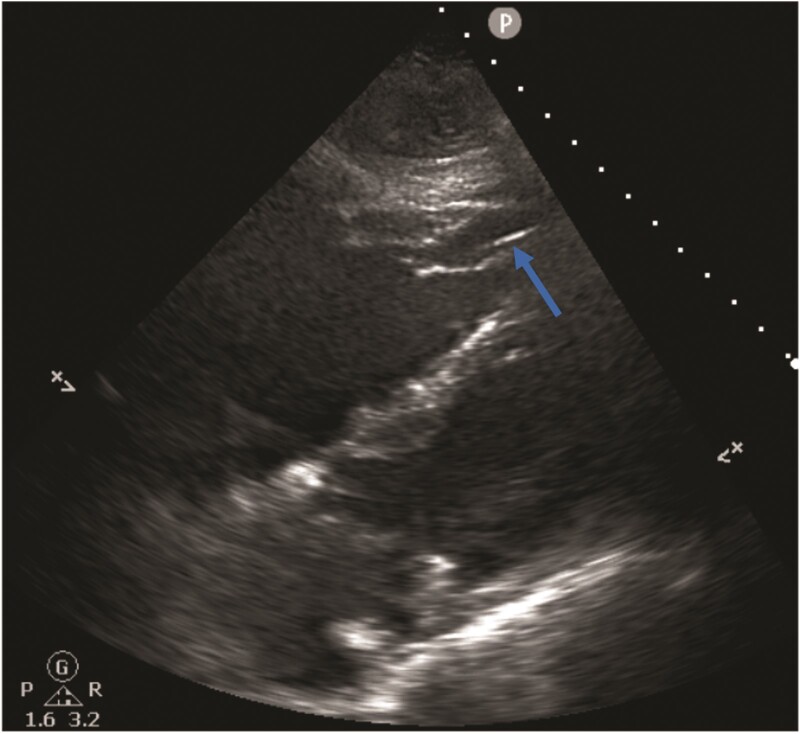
Subcostal view on echocardiogram demonstrating part of the pacing lead in the right ventricular apex (arrow). No pericardial effusion is seen.
